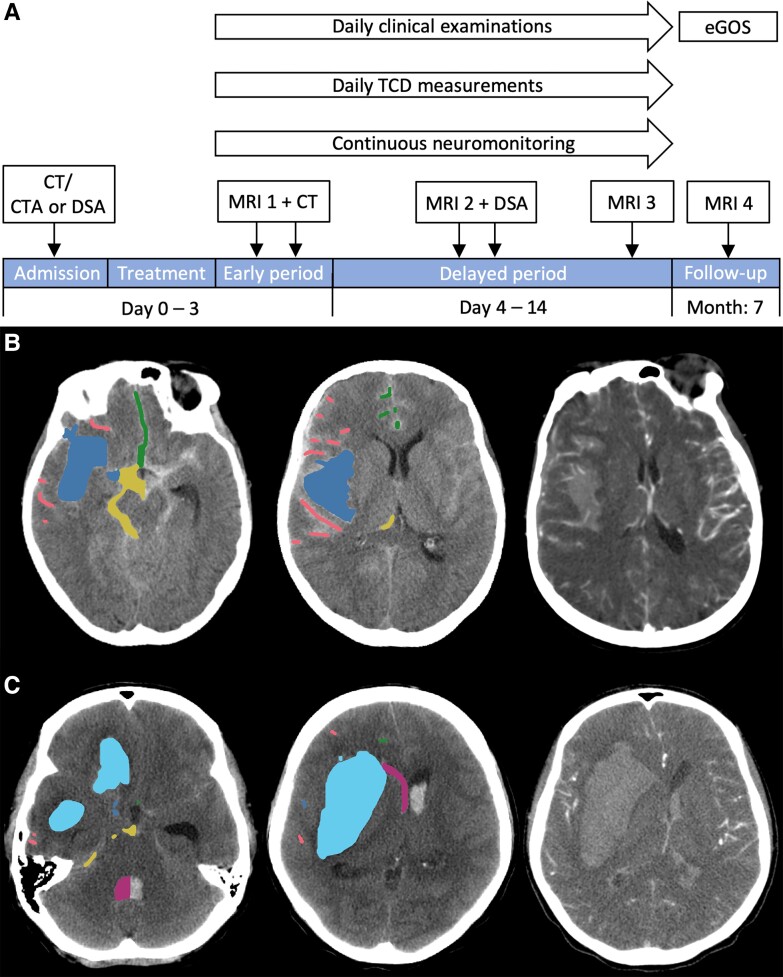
Diagnostic flow of the DISCHARGE-1 study and quantification method for ipsilateral haemorrhage on the initial CT scan according to six predefined compartments. (A) CT and CTA were performed on admission. If necessary, CTA was complemented by DSA. The first MRI (MRI 1) was acquired 24–48 h after surgical or endovascular treatment of the aneurysm. In addition, a postoperative CT was performed to locate the subdural electrodes. In the delayed period, MRI 2 was performed around Day 7 and MRI 3 around Day 14. Note that delayed infarct volumes were quantified for both sessions separately and then added up for further analysis. A second DSA was performed around Day 7 to assess angiographic vasospasm. After treatment of the aneurysm, the patient was transferred to the neurocritical care unit, where continuous neuromonitoring, daily TCD and clinical examinations started and continued until Day 14. After 7 months, a follow-up MRI 4 was performed. Furthermore, functional outcome was documented using the extended Glasgow Outcome Scale (eGOS). (B) Representative CT and CTA images of a patient with aSAH from a right MCA aneurysm. Note that haemorrhage was only quantified in the hemisphere ipsilateral to the subdural electrodes. CTA (right image) demonstrated contrast-enhancing vessels inside a large right-sided haematoma. Therefore, the volume of this haematoma was quantified according to the category of subarachnoid blood in the Sylvian fissure (blood_Sylvian_) (blue label in left and middle image). Blood_convex_ comprised blood in the sulci at the cerebral convexity including the rami of the Sylvian fissure (red label). Blood_inter_ was composed of blood in the anterior and posterior interhemispheric fissure as well as adjacent sulci (green label). Interhemispheric blood that crossed the midline was classified as contralateral and was not considered. Blood_basal_ included blood in the following cisterns: prepontine, interpeduncular, suprasellar, ipsilateral ambient, quadrigeminal and the interpositum cistern (yellow label). Subdural blood, seen as narrow hyperdense fringe overlying the left frontal cortex, was not quantified. (C) Representative CT and CTA images of another patient with aSAH from a right MCA aneurysm. CTA (right image) demonstrated contrast-enhancing vessels of the M2 segment of the MCA outside a large right-sided haematoma. Therefore, this haematoma volume was quantified within the ICH category (cyan label in left and middle image). Blood in the ventricular system is shown in purple. Most notably, a large clot was found in the fourth ventricle. This was only quantified until the midline (purple label). Blood_basal_ (yellow label), blood_inter_ (green label) and blood_convex_ (red label) were small in this patient.
